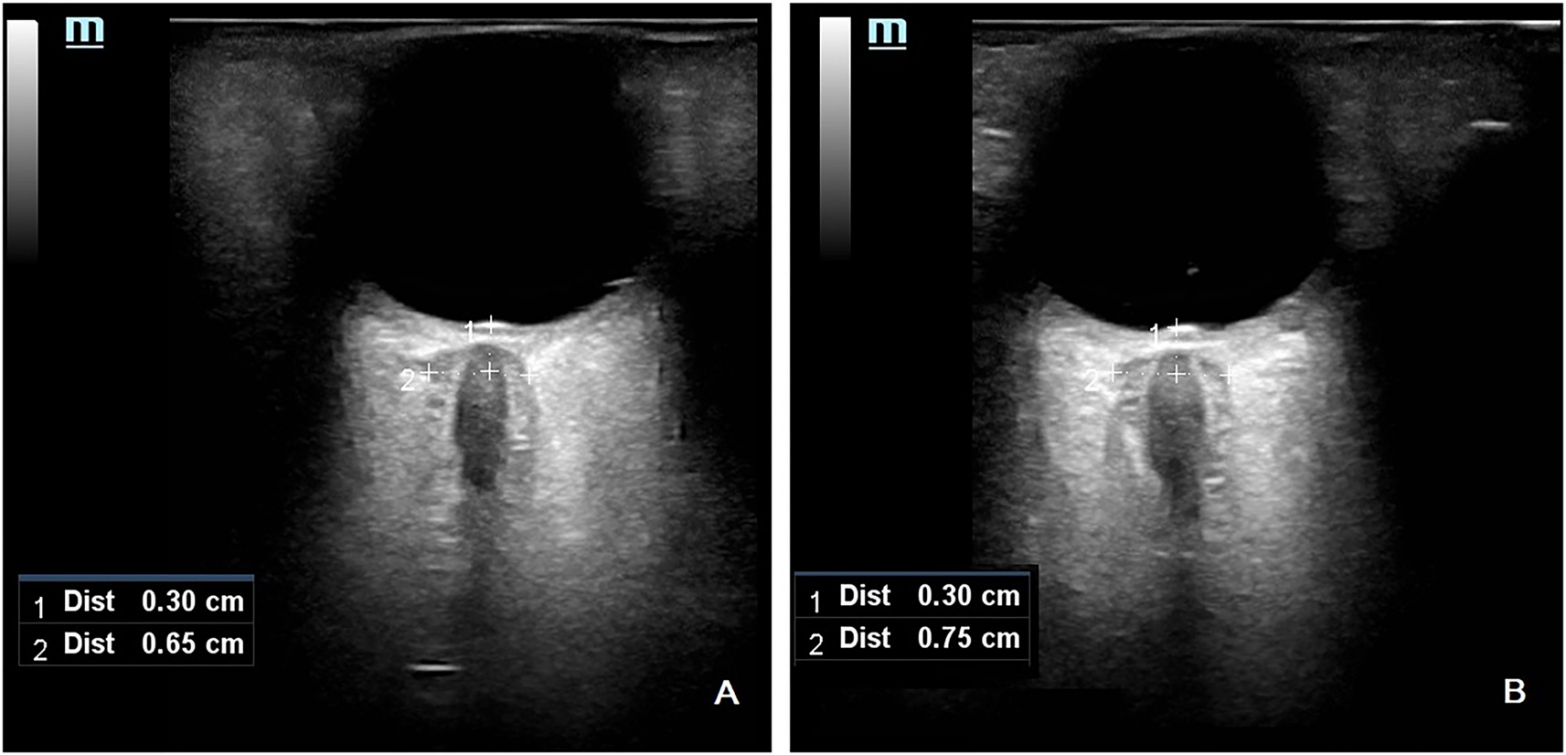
Ultrasonographic ONSD measurement. (A) Ultrasonographic ONSD measurement of patient number 11; (B) Ultrasonographic ONSD measurement of patient number 32. ONSD: Optic nerve sheath diameter.
